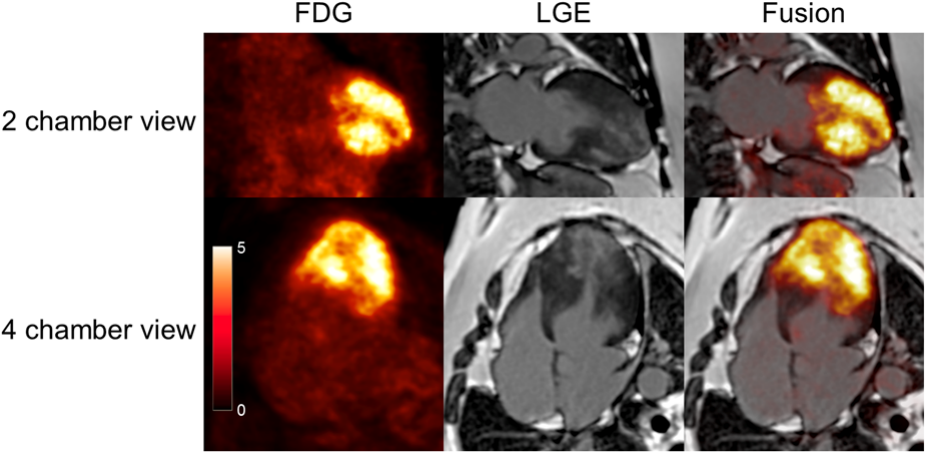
^18^F-fluorodeoxyglucose PET/MRI images of 2- and 4-chamber views. Intense ^18^F-fluorodeoxyglucose (FDG) uptake was observed on the middle walls in the left ventricular myocardium. Late gadolinium enhancement (LGE) was detected on the apex. Fusion images clearly showed the markedly increased FDG uptake in hypertrophied myocardial regions
